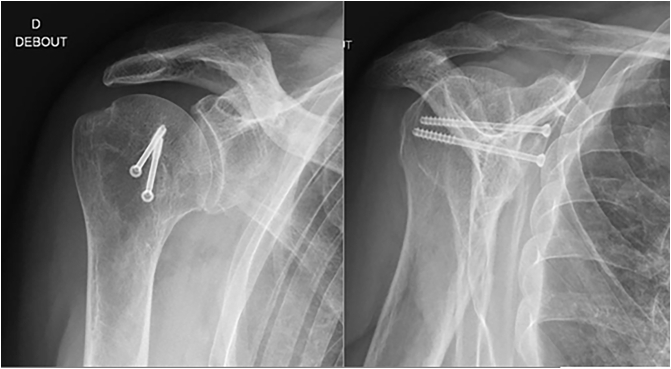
14 months follow-up X-ray Right shoulder.
